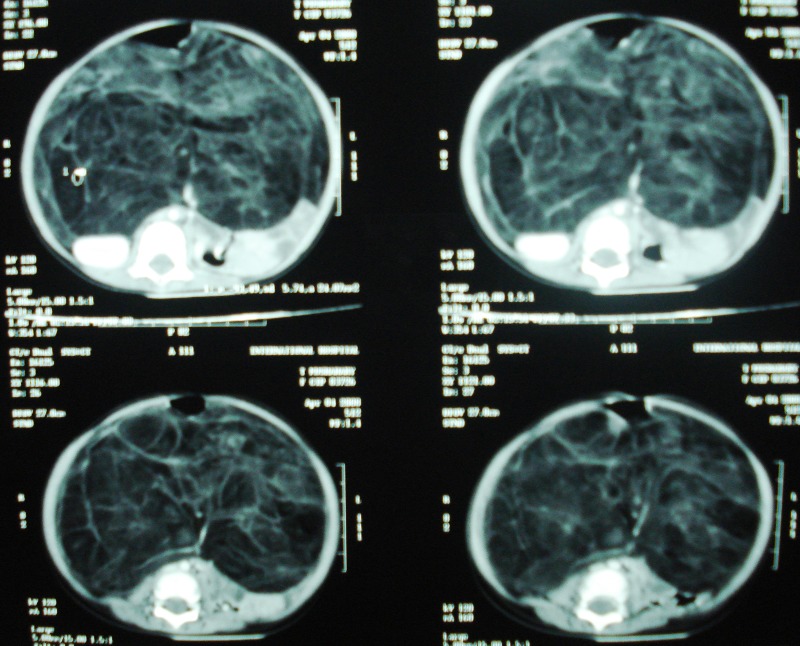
Figure 1:CT scan showing retroperitoneal mass, kidneys are seen separately
